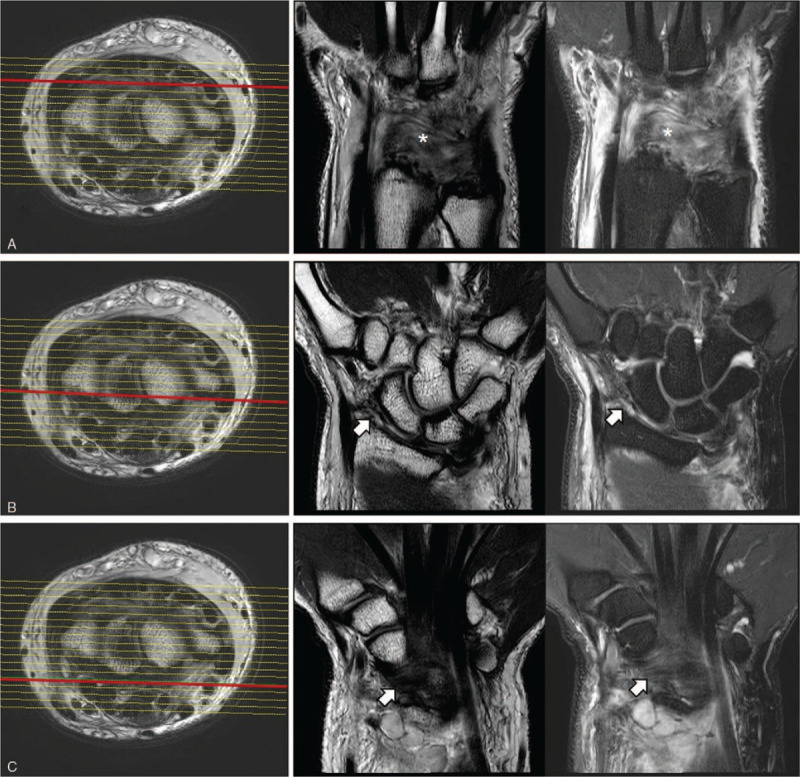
Preoperative MRI scans. (A) Disruption of the dorsal ligaments and capsules, (B and C) avulsed from the proximal insertion of the volar radiocarpal ligaments. MRI = magnetic resonance imaging.
